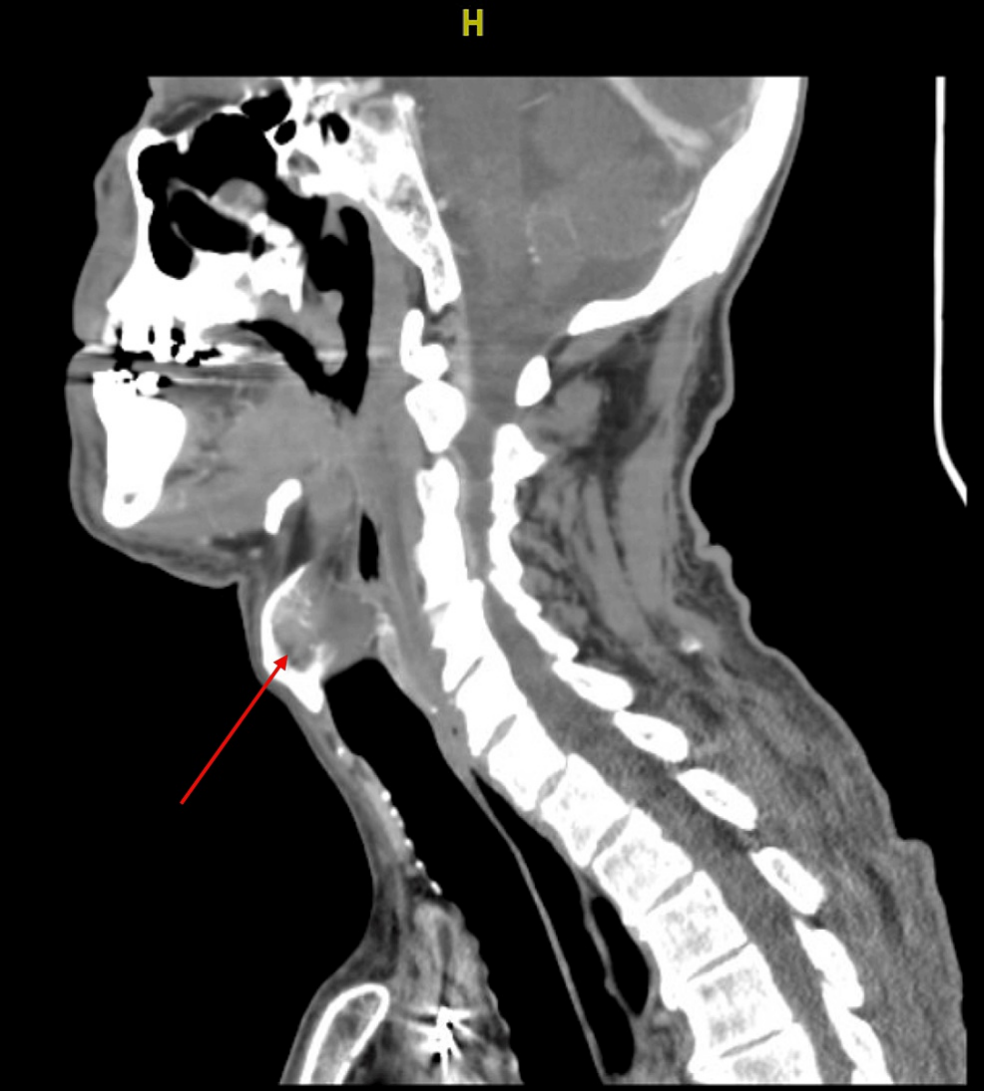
Sagittal CT of the head and neck This figure shows how the left hypopharynx extends into the left pre-epiglottic vallecula where there is an ill-defined soft tissue mass (red arrow). There is diffuse bulky centrally necrotic conglomerate lymphadenopathy throughout the bilateral cervical chains.
